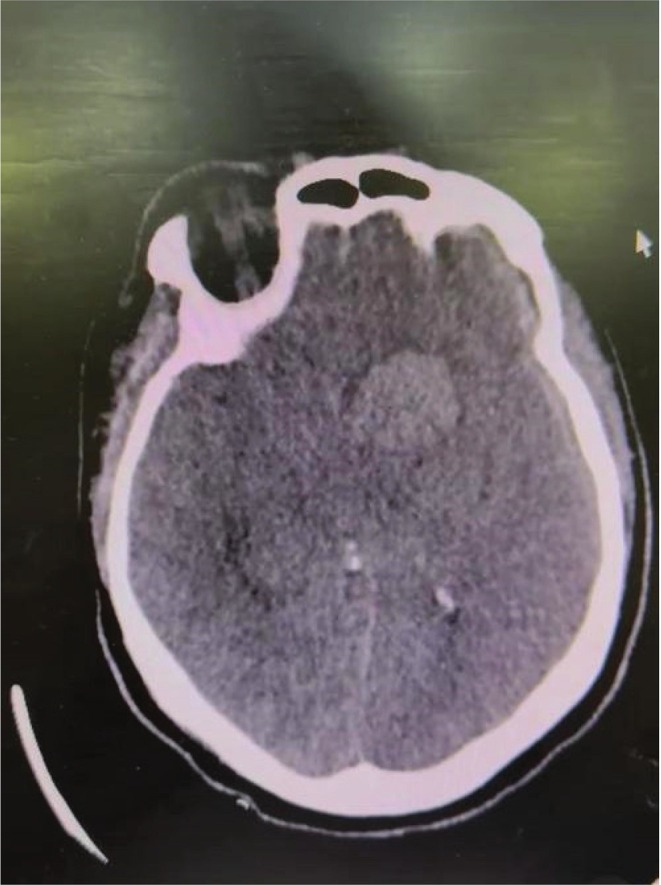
Patient's brain CT scan that showed a subarachnoid hemorrhage (SAH) with a left subdural hematoma.
